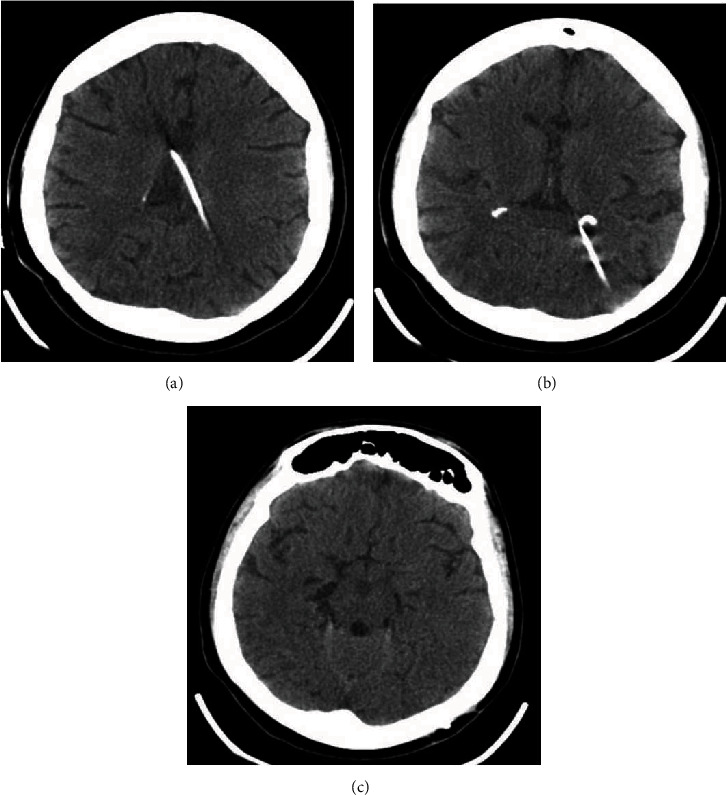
(a–c) Postoperative cranial CT demonstrating the reduction of ventricle sizes. The ventricle catheter is well positioned.
